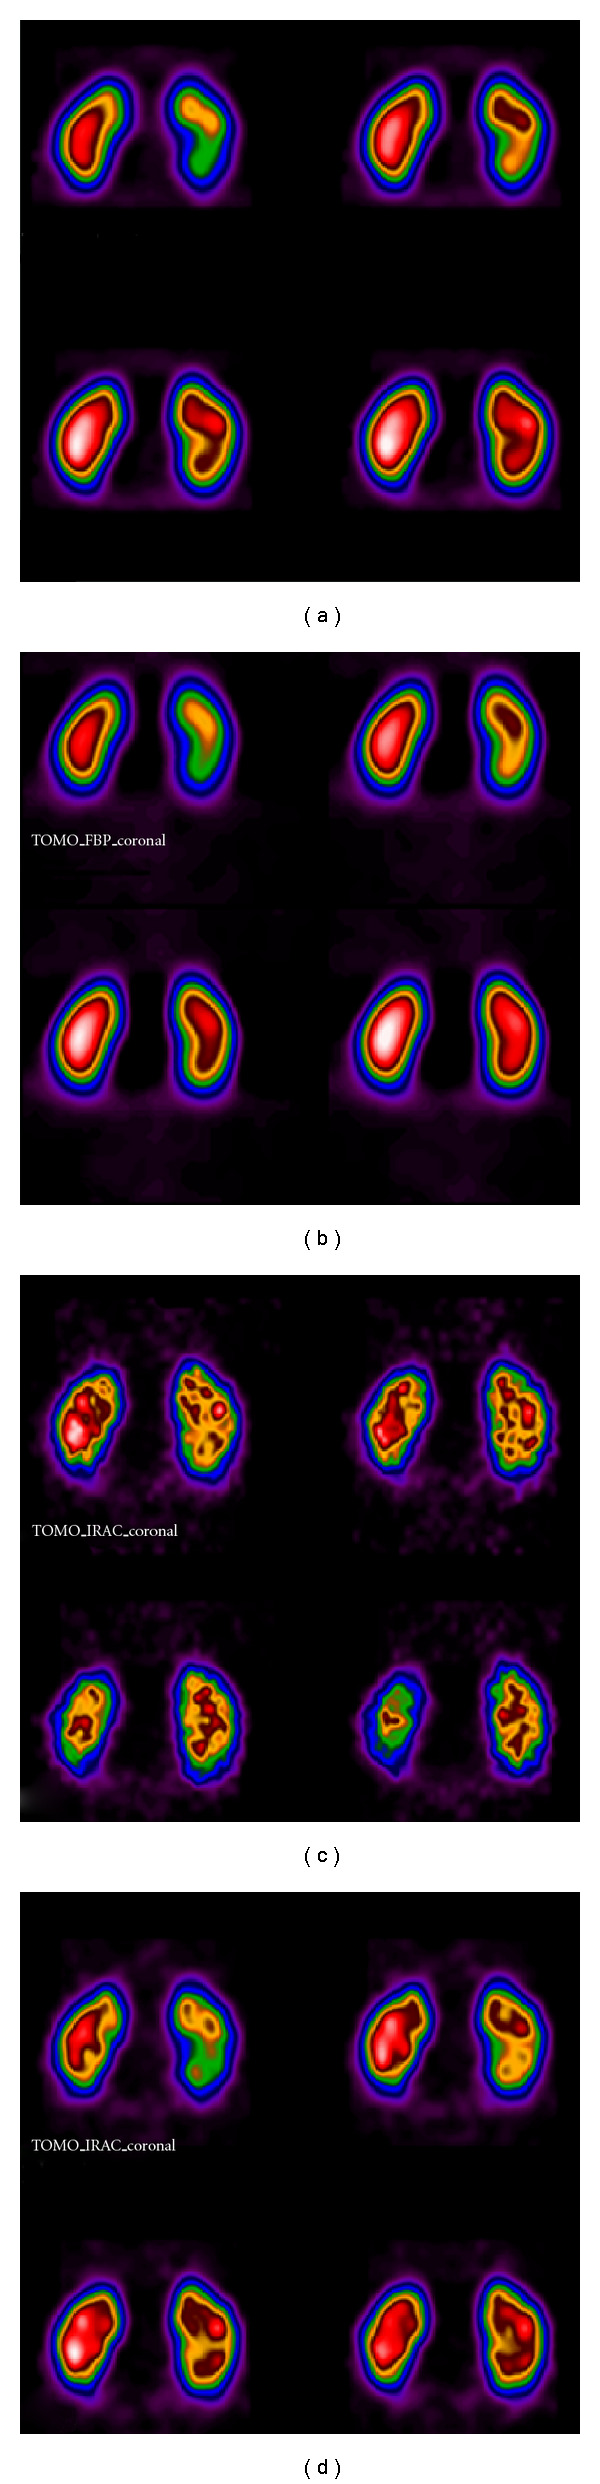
Coronal slices of renal SPECT study. The effect of filtering in smoothing and contrast of SPECT reconstruction, by FBP and Chang attenuation correction (coefficient 0.11) or OSEM iterations is reflected on clinical images. (a) FBP reconstruction, Butterworth filter (critical frequency 0.5 cm^−1^, power 10) (b) FBP reconstruction, Hann filter (critical frequency 0.9 cm^−1^) (c) OSEM 10 subsets/10 iterations (d) OSEM 10 subsets/10 iterations and postfiltering by Butterworth (critical frequency 0.5 cm^−1^, power 10).* Study has been completed in Radiation Physics Unit, Department of Radiology, University of Athens*.
